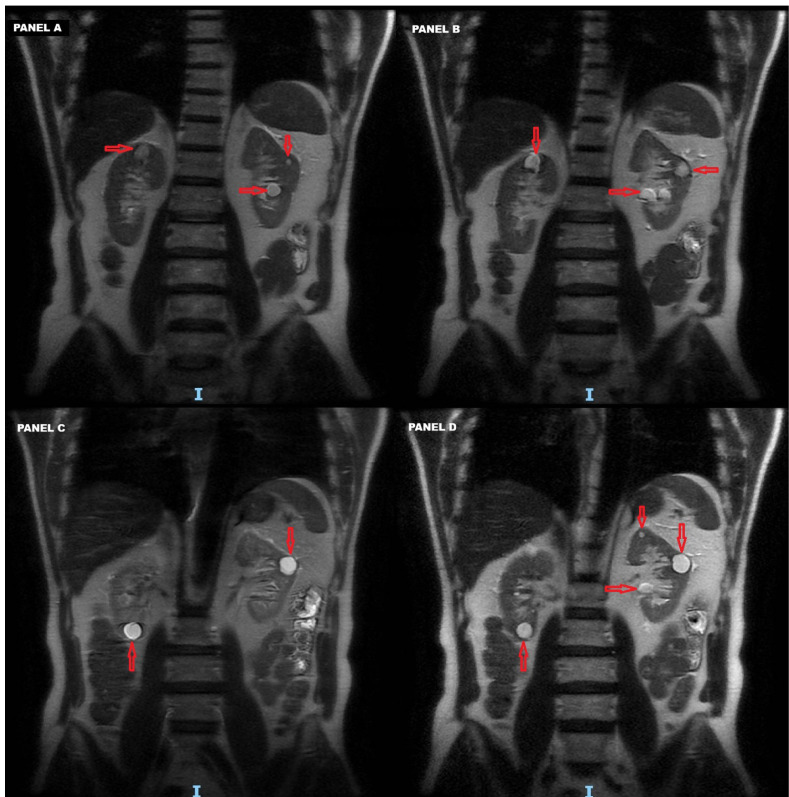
Abdominal magnetic resonance imaging performed in 2021 showing bilateral cortical and medullary cysts at renal level. A-D. Serial sections showing the progressive presence of renal cysts in a depth-wise scan. Craniocaudal description: Right kidney: upper pole 17 mm, middle third 7 mm, lower pole 17 mm. Left kidney: upper pole 6 mm (x2), middle third 17 mm and 6 mm, lower third two sinus cysts of 18 mm and 16 mm. Red arrows: location of the renal cysts.
